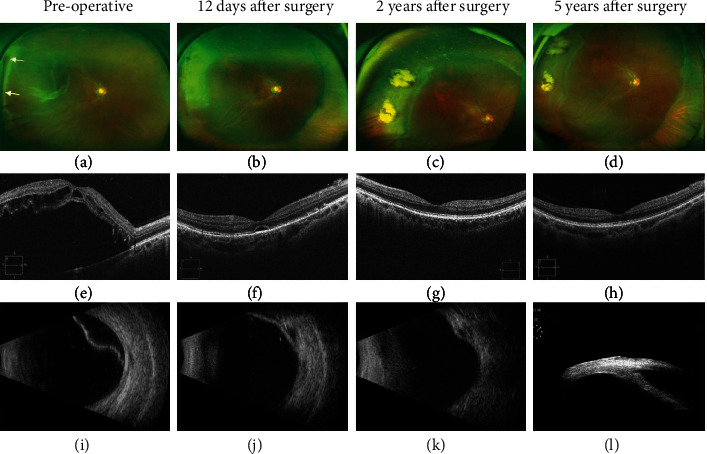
Examination result of case 10 before and after surgery. (a) Preoperative fundus photography showed retinal detachment and holes. (b)–(d) Fundus photography showed retinal reattachment, scleral buckle ridge, and cryoretinopexy scar at different times after surgery. (e) OCT demonstrated macular off and intraretinal cyst before surgery. (f)–(h) OCT showed macular reposition. (i) B-ultrasound confirmed retinal detachment. (j, k) B-ultrasound demonstrated retina reattachment and scleral buckle ridge. (l) 25-gauge chandelier insertion site was checked by UBM.
